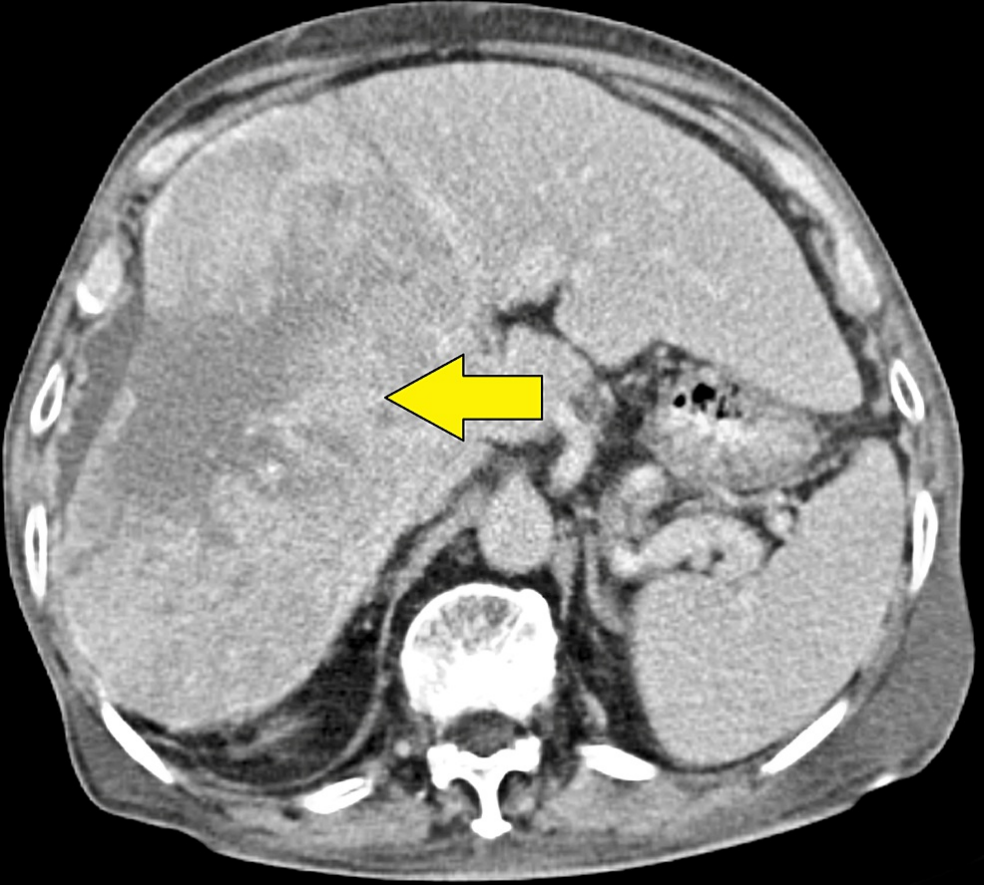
Computed tomography revealed a hypoechoic lesion in the right lobe of the liver (indicated by yellow arrow) with ascites
